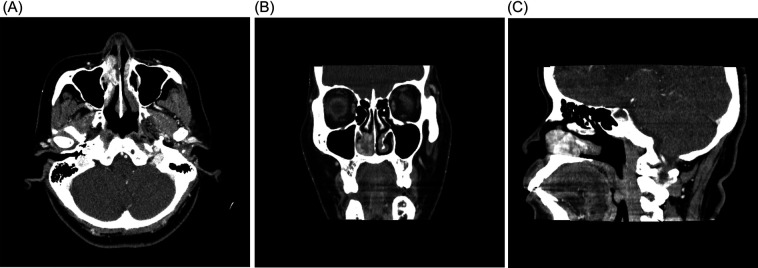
Preoperative imaging studies. Contrast-enhanced CT (A) axial, (B) coronal, and (C) sagittal views showed an enhancing mass originating from the right inferior turbinate, extending medially toward the nasal septum and posteriorly to the middle of the nasal cavity at the level of the inferior meatus.
